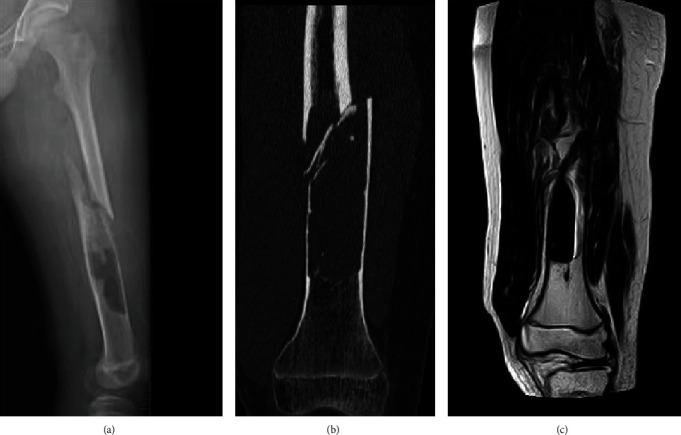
Imaging manifestations of myelolipoma. (a) X-ray showed a pathological fracture of the left femoral shaft. (b) CT showed the disappearance of bone trabeculae at the tumor and thin cortex. (c) MRI showed thinning of the bone cortex in the middle and lower segments of the left femur and patchy low signal shadow in the medullary cavity.
